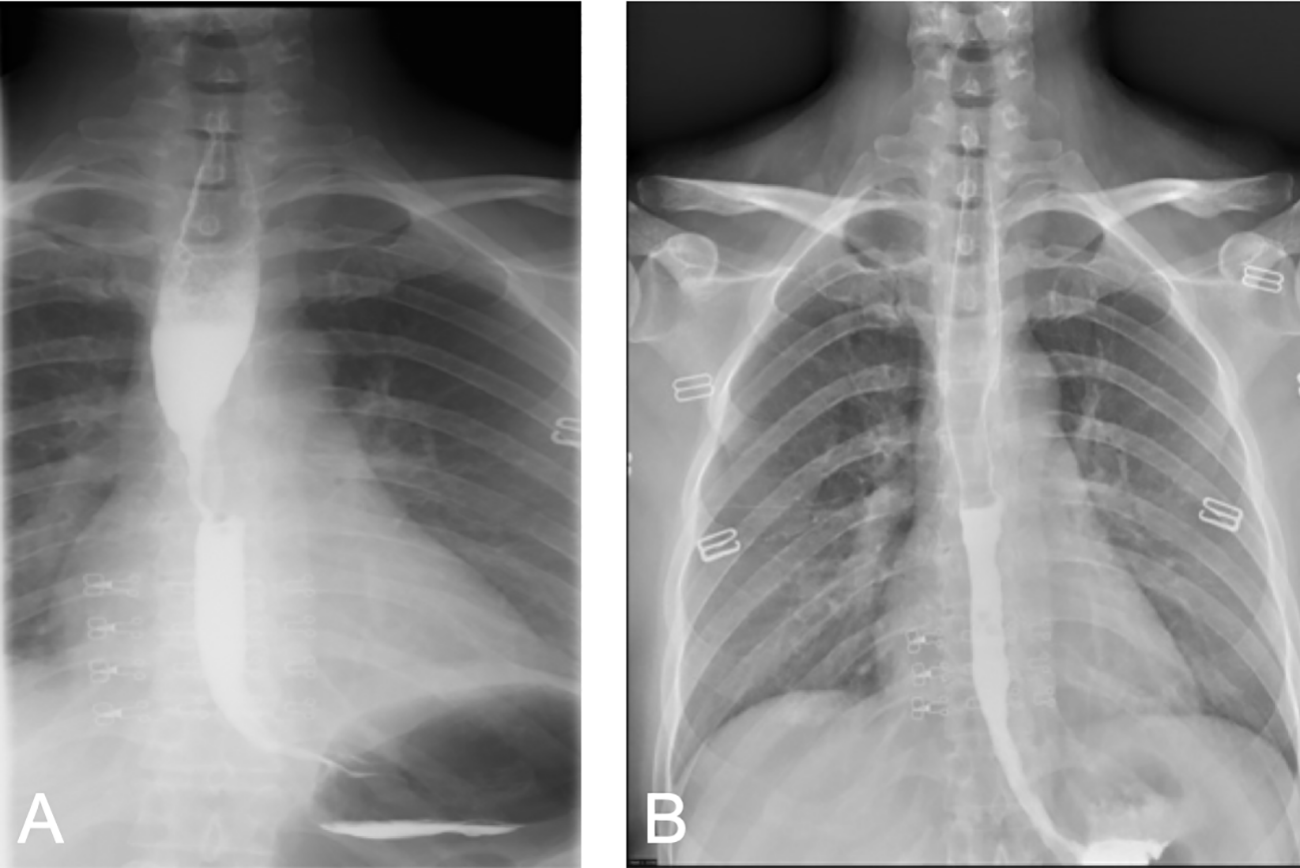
Comparison of barium swallow radiography before and after treatment. (A) Before treatment, localized narrowing of the esophageal lumen, involving an area of approximately 38 mm, with dilation above the narrowed segment. (B) After treatment, the local esophageal wall mucosa appears slightly irregular.
